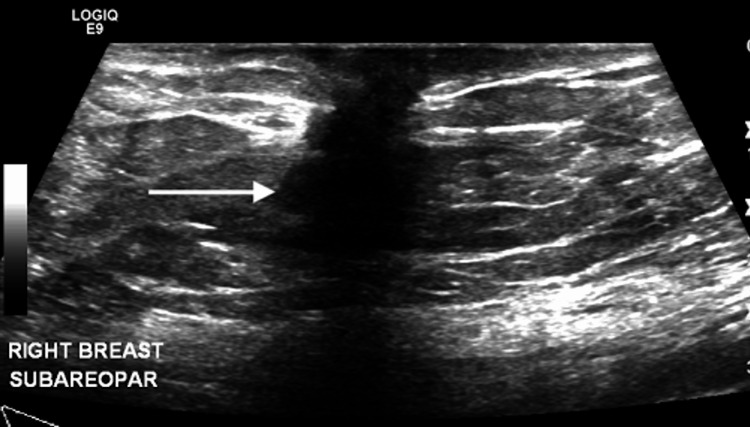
Survey ultrasound of the right breast and axilla demonstrates a 1.4 x 1.3 x 1.2 cm solid, hypoechoic, and irregular mass at the subareolar region. The remainder of the breast and axilla examination is unremarkable.
